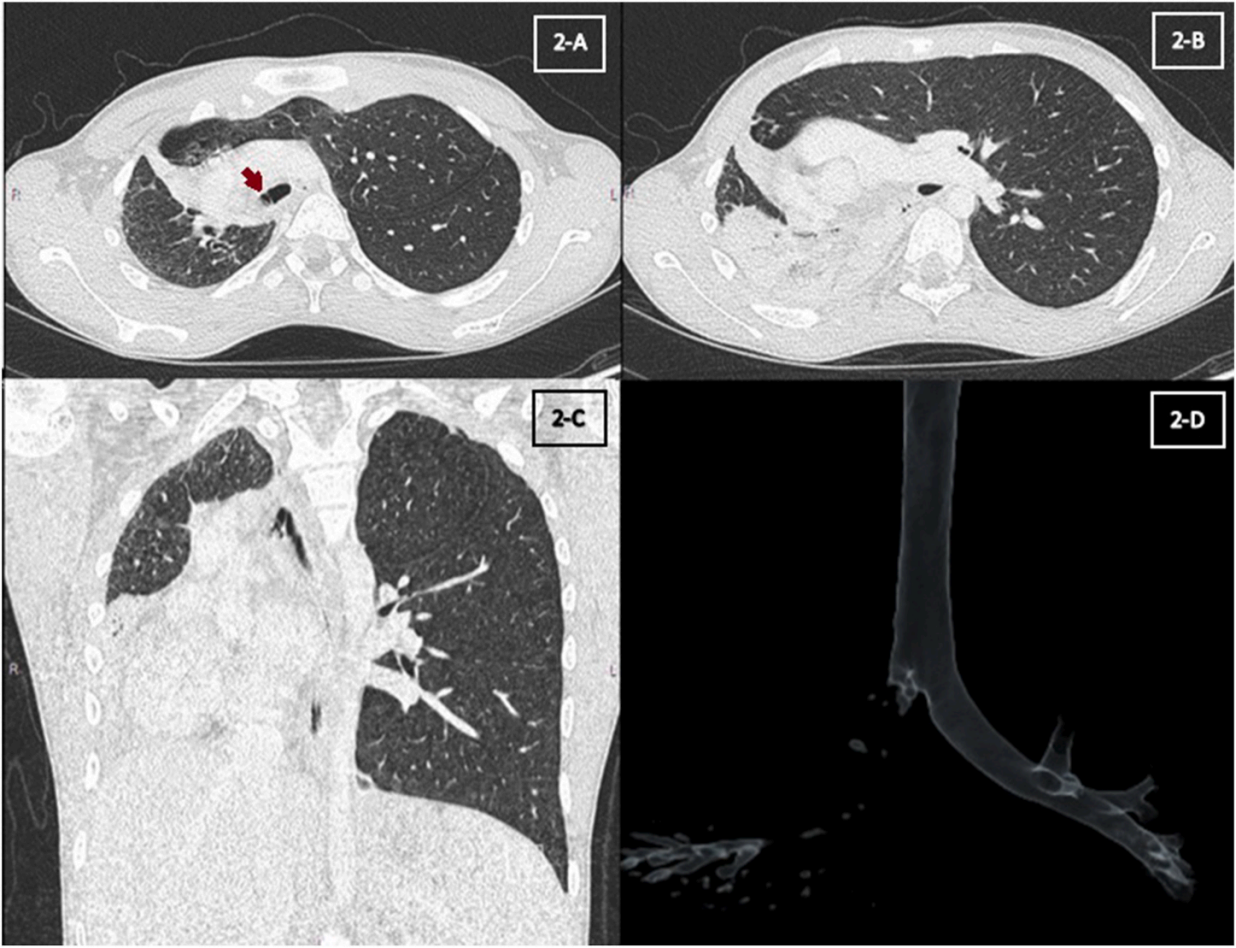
(A) Chest Computed Tomography - Transverse section(Red arrow shows bronchial web) (B) Chest Computed Tomography - Transverse section showing right lobar collapse (C) Chest Computed Tomography - Coronal section (D) Chest Computed Tomography - Three-dimensional image of the airways. (For interpretation of the references to colour in this figure legend, the reader is referred to the web version of this article.)
